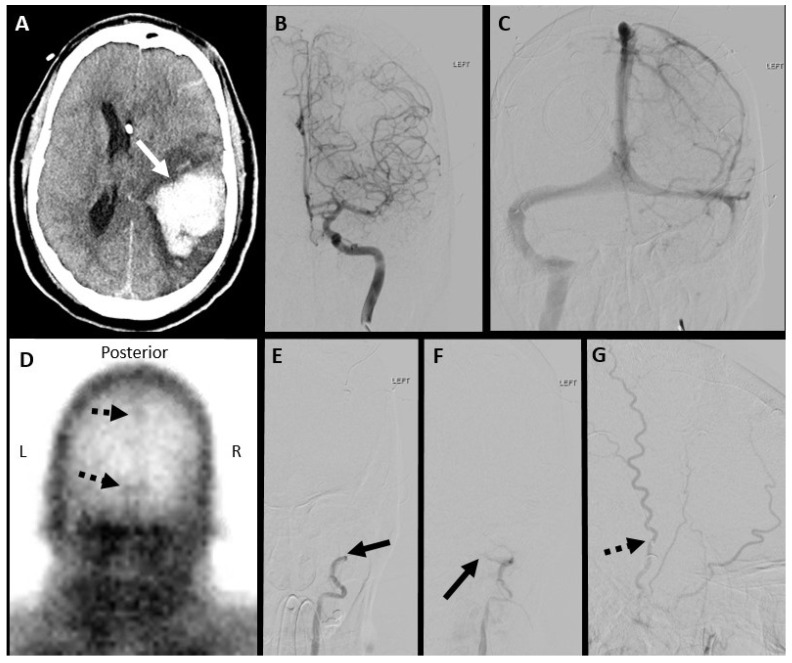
Fifty-four-year-old female with BD/DNC in the clinical deterioration of intracranial hemorrhage. (A) Unenhanced axial CT brain at the initial presentation demonstrates parenchymal hemorrhage in the left fronto-parietal lobe with mass effect (solid white arrow) with associated subarachnoid hemorrhage. (B) Arterial and (C) venous phase of carotid angiogram at the initial presentation does not demonstrate active contrast extravasation with patent intracranial arterial and venous circulation. Patient clinically deteriorated over a period of 7 days with clinically absent brain stem reflexes. (D) Faint intracranial midline tracer activity on Tc-99m DTPA nuclear scintigraphy favoring the inconclusive diagnosis of the BD/DNC (dashed black arrows in (D)). (E–G) Follow-up catheter angiography was performed, and it confirms the diagnosis of BD/DNC where nuclear scintigraphy was inconclusive. There is the non-visualization of internal carotid artery beyond the petrous segment (solid black arrows in (E,F)) with the contrast opacification of branches of external carotid arteries (dashed black arrow in (G)).
